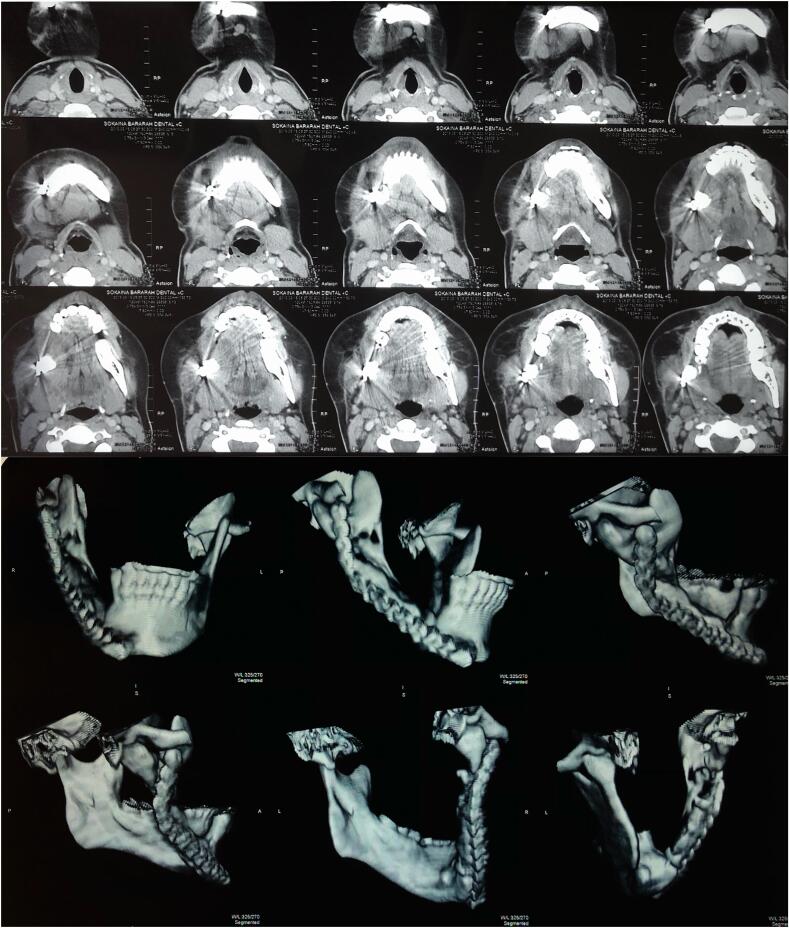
Post-operative CT and CBCT shows reconstruction plate in the region of excision.
